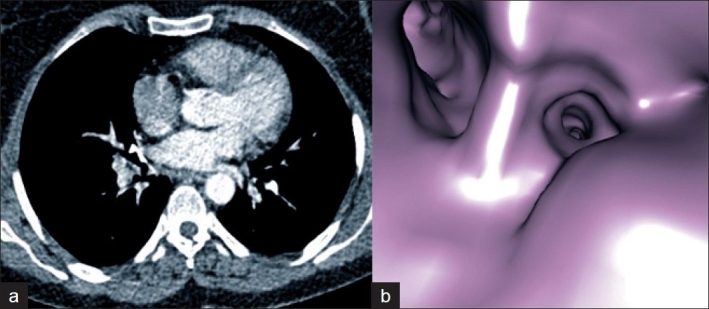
Chronic inflammation case a, b - Axial CT and VB images of the external compression due to calcified lymphadenopathy on the antero-medial wall at the entrance of the right middle lobe. This case was reported by FOB as an obstruction in the right medial middle lobe
